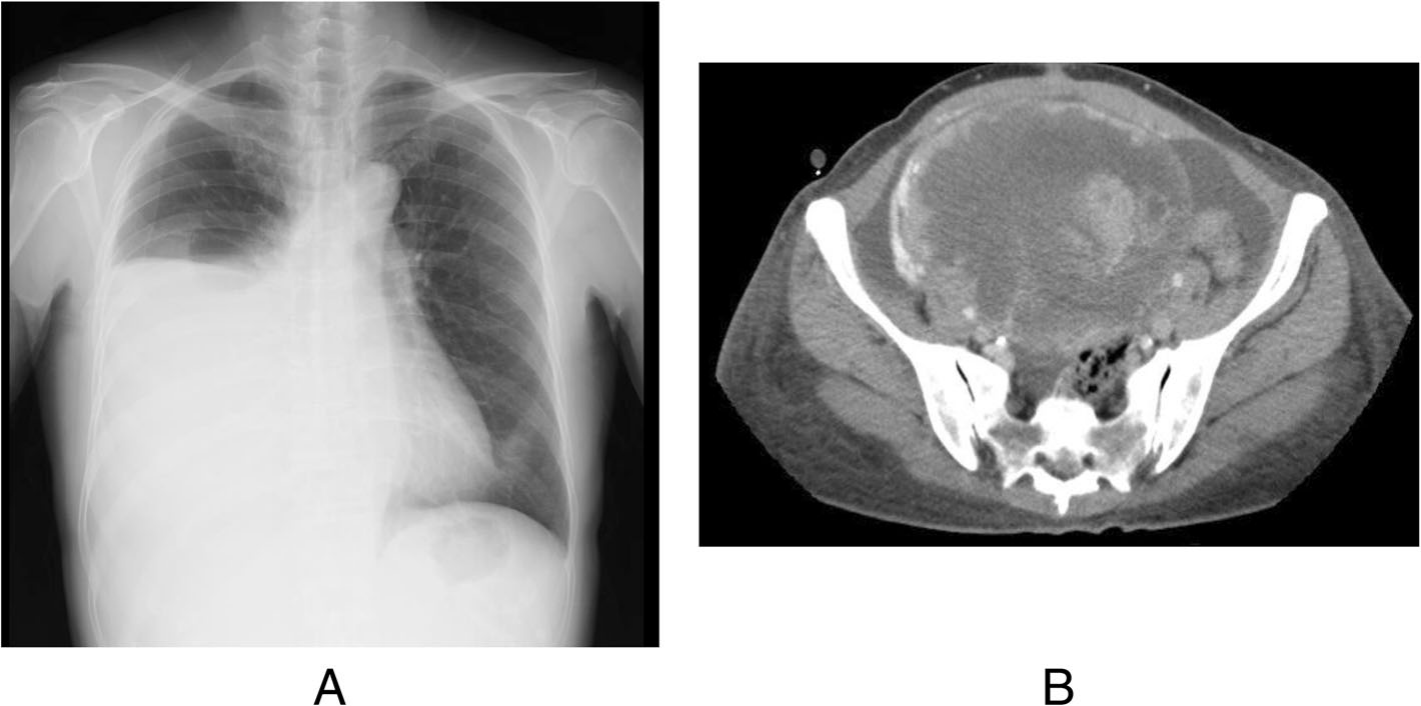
a Six months after the operation, pleural effusion of the right side has developed again. b CT revealed huge ovarian tumor again
